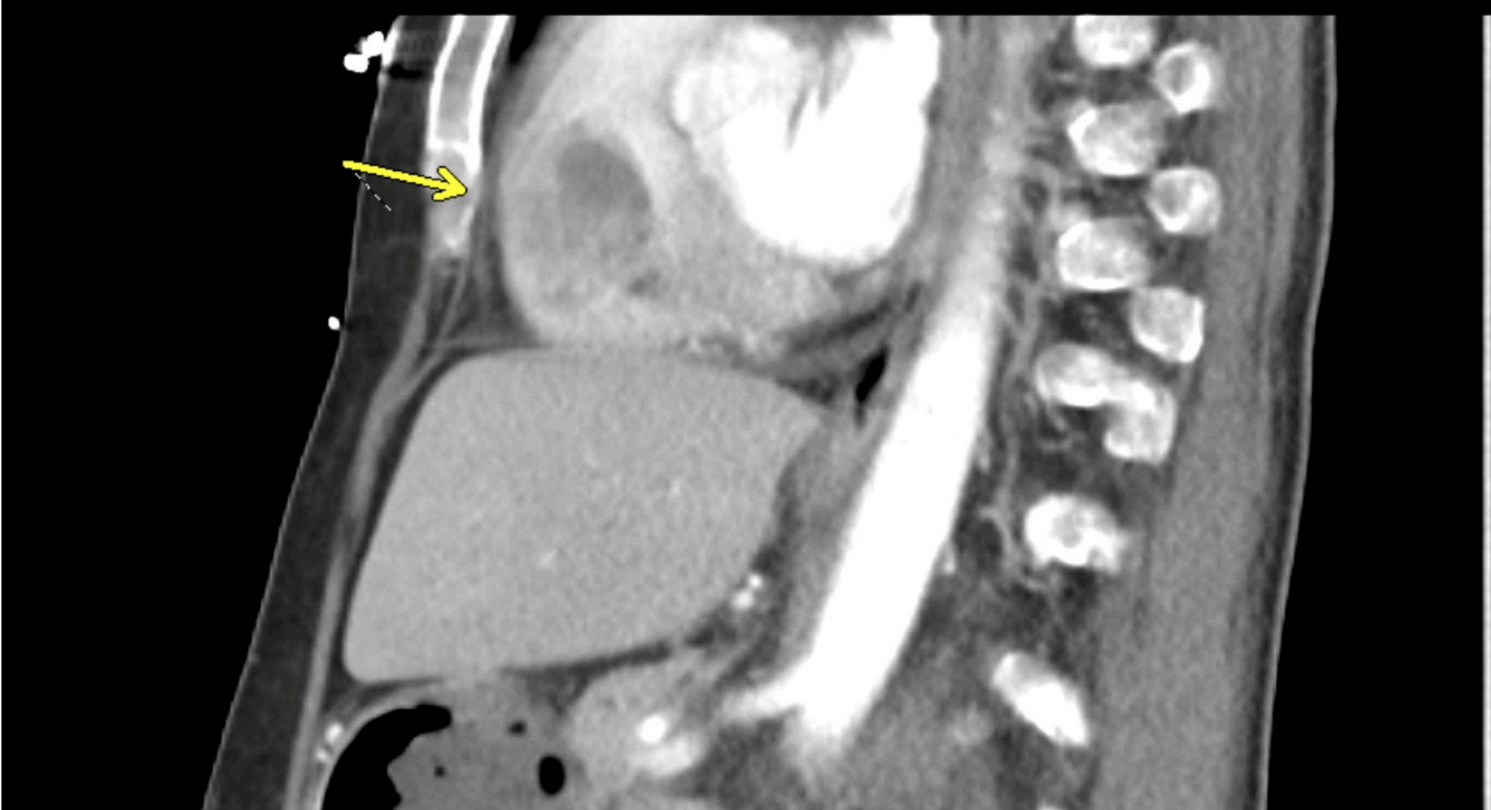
Initial sagittal post-contrast CT chest showing right ventricular filling defect (arrow).
